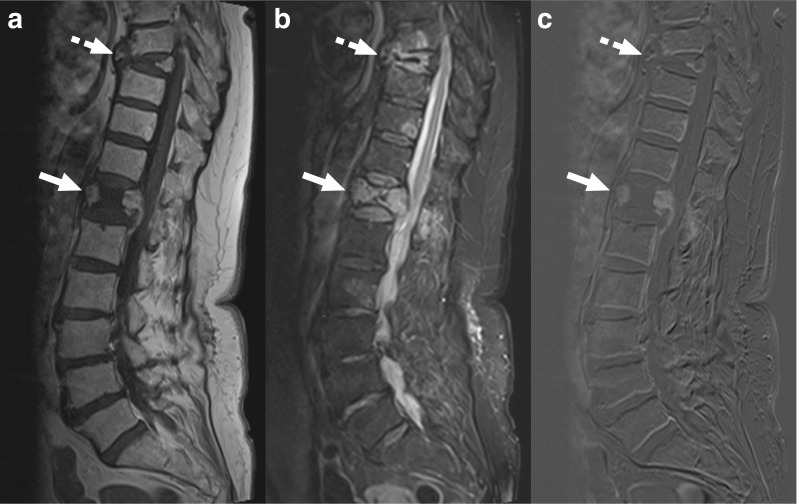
An 80-year-old woman with MRI findings consistent with osteoporotic vertebral fractures. Sagittal T1-weighted (a), fat-suppressed T2-weighted, and gadolinium-enhanced subtraction images (c) show collapse of vertebrae T12 (*continuous arrows*) and T8 (*dashed arrows*), with edema (b) and gadolinium enhancement of anterior and posterior portions of the T12 vertebra (c), and the impression of some edema in adjacent discs (b). However, there is no clear involvement of two consecutive vertebrae. Moreover, the involvement of multiple levels and the configuration of the affected vertebrae strongly suggest osteoporotic vertebral fractures. This patient underwent CT-guided biopsy with spondylodiscitis in the differential diagnosis of the original clinical report, but was excluded from this study
